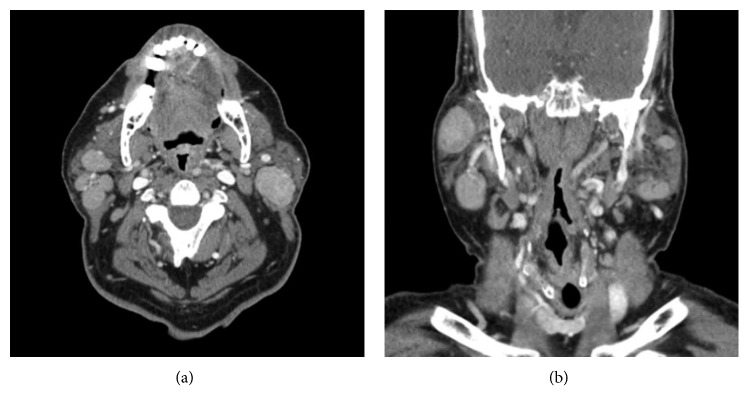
Computed tomography (CT) with contrast in axial and coronal planes showing bilateral parotid lesions and cervical lymphadenopathy.
